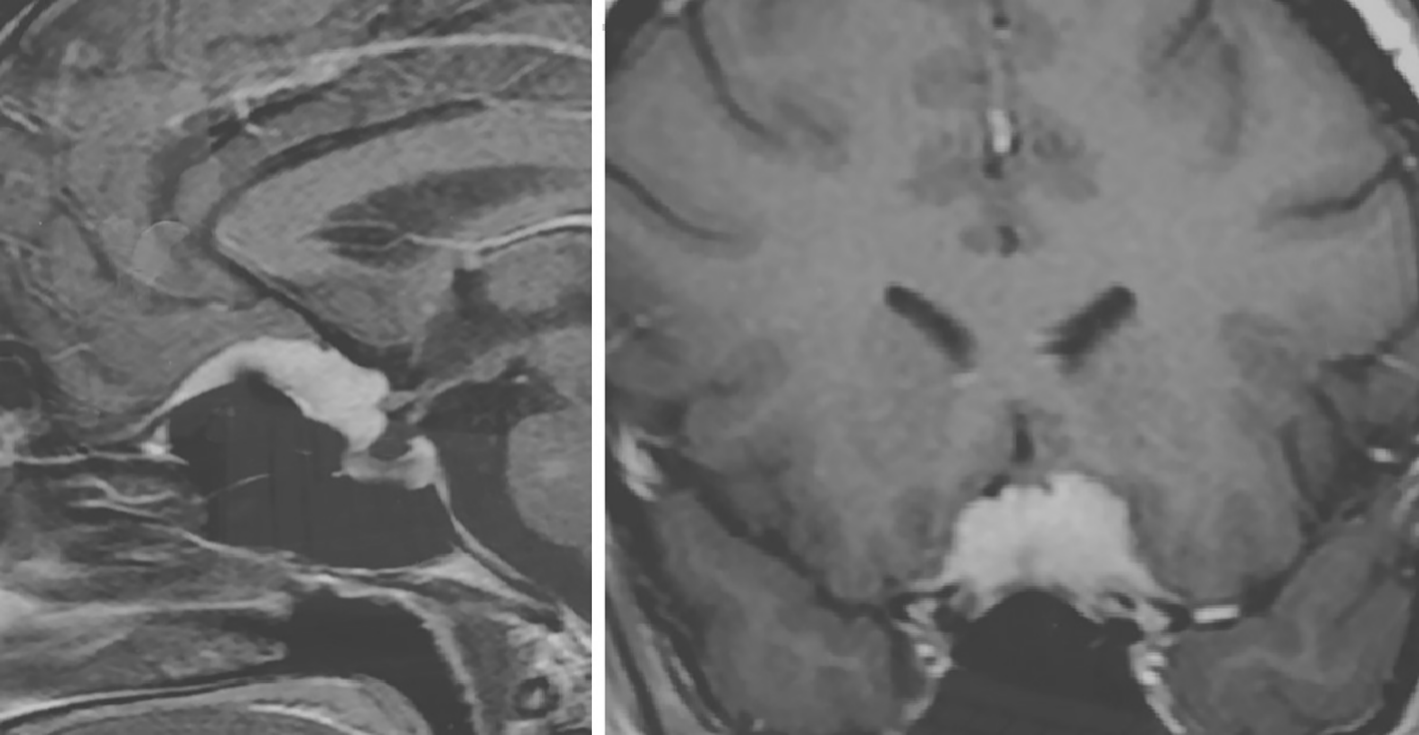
Post-contrast cranial MRI of 35-year-old female with tuberculum sellae meningioma presenting sudden onset of visual deficit during artificial *in vitro* fertilization treatment on the therapy with human chorionic gonadotrophin.
